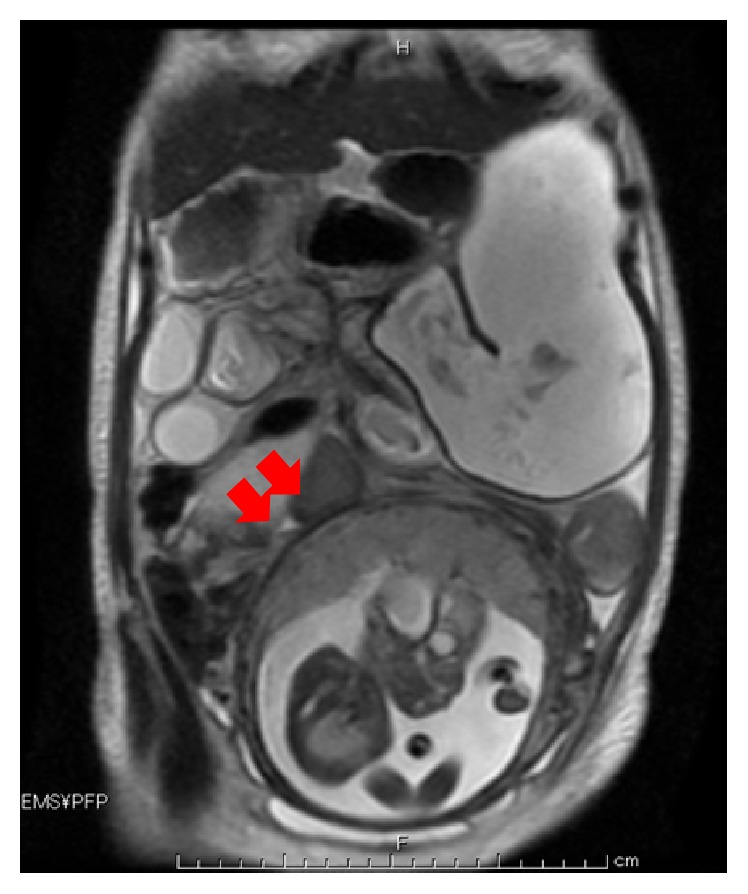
MRI showing the narrowing of the distal extent of the ileum and the dilated proximal intestine (arrows).
